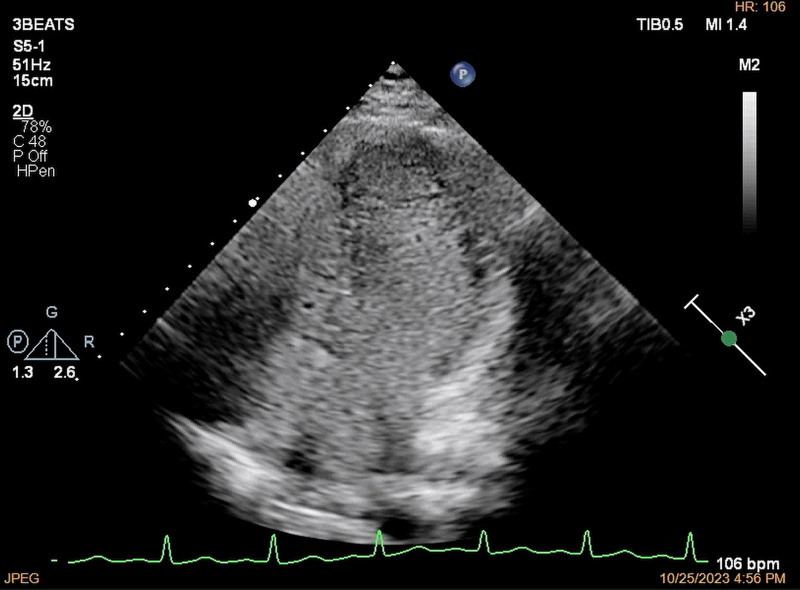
The apical 4-chamber view showed that a large number of microbubbles were found at the left-sided heart chambers simultaneously after the effusion of the right-sided heart chambers by agitated saline contrast transthoracic echocardiography through right medial malleolus vein.
